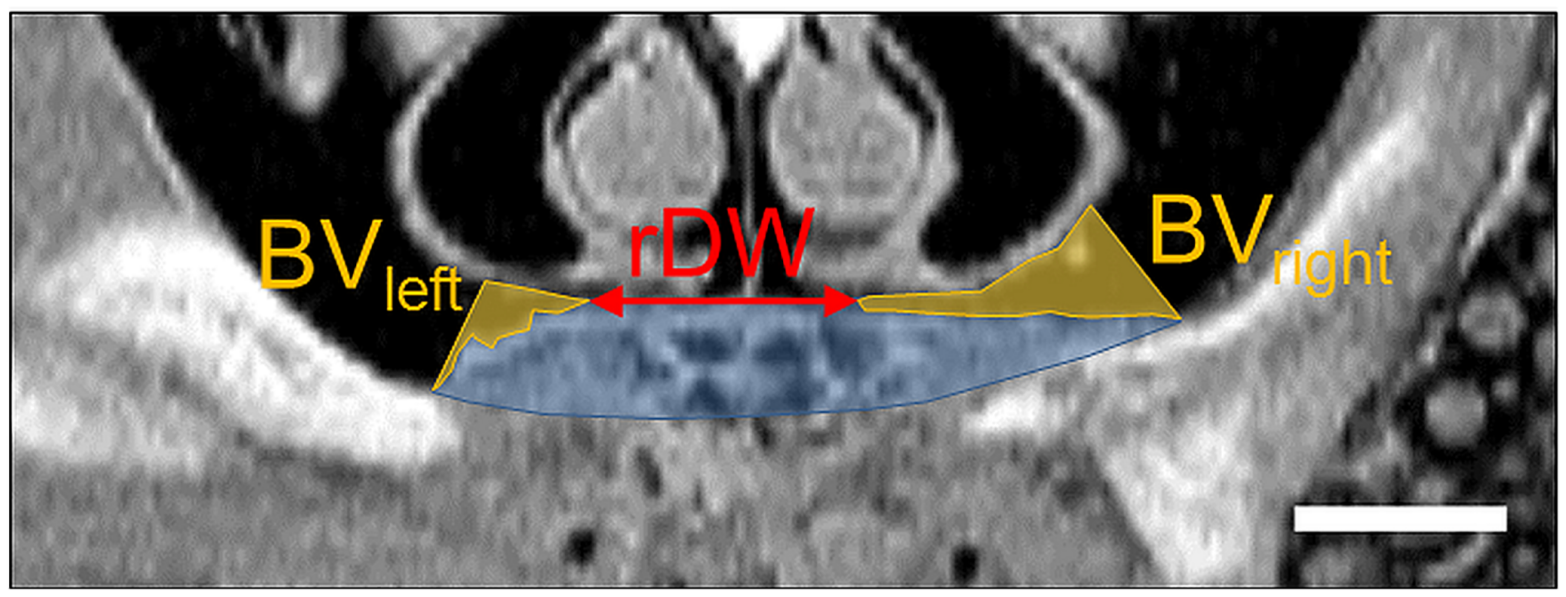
Measured parameters used for the concordance analysis. Here, a MRI slice image of a control was shown exemplarily. A detailed description of the tissue structures is given in Fig 3 and Table 3. To determine the overall newly formed bone (*BV*), the bone tissue area at the left and the right side of the artificial defect was measured. The remaining defect width (*rDW*) is marked with the red arrow. The scale bar represents 1.0 mm.
